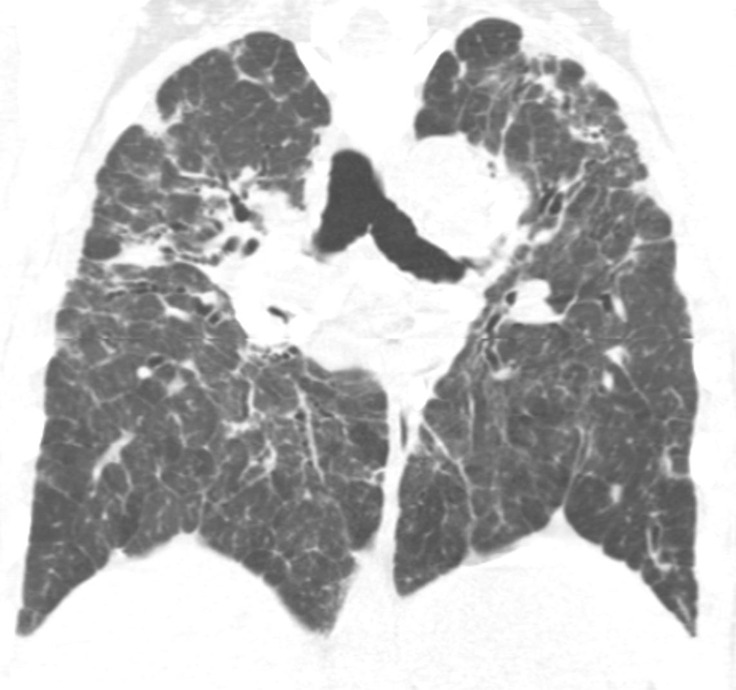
Advanced fibrotic sarcoidosis. (a–c) Axial inspiratory CT and (d) coronal inspiratory CT images demonstrate mid to upper lung–predominant pulmonary fibrosis with marked architectural distortion, associated lung nodularity, and some mosaic attenuation. Note also the calcified mediastinal lymph nodes. This case would be categorized as most consistent with a nonidiopathic pulmonary fibrosis diagnosis based on these features.
